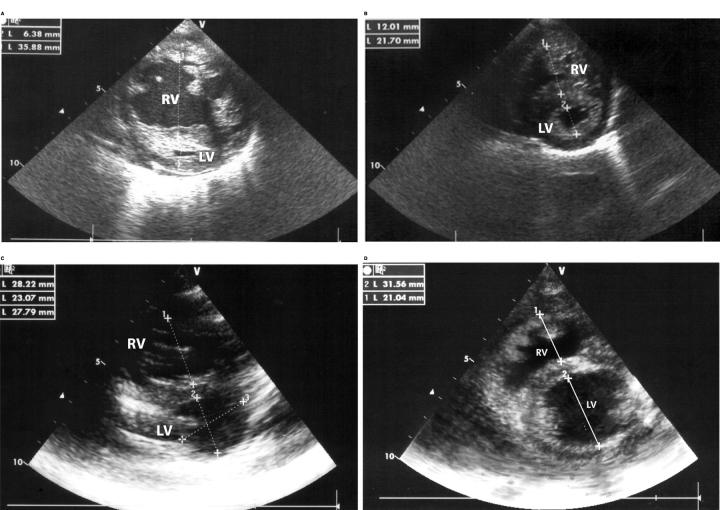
Patient 22 in Table 1, initially in FC 3, suprasystemic PH and 38.7 WU Resistances, with positive answer to the O2Test and hyperreactivity of pulmonary vasculature. (A, B) Initial Echo (1 years old). Short-axis view of the ventricles in Systole (A) and Diastole (B). These views show a very small left ventricle mainly in Systole. (C) (Systole) and (D) (Diastole): Current echocardiogram. Short axis view of the ventricles 6 years after treatment and in FC 1. The diameter of the left ventricle is much bigger in systole and diastole in relation with the first echocardiogram. The RV/LV relation changed significatively. This case describes some aspects: The importance of early detection of PH, the importance of hyperreactivity of pulmonary vasculature at early age and the positive changes in few years. RV, right ventricle; LV, left ventricle; PH, pulmonary hypertension.
